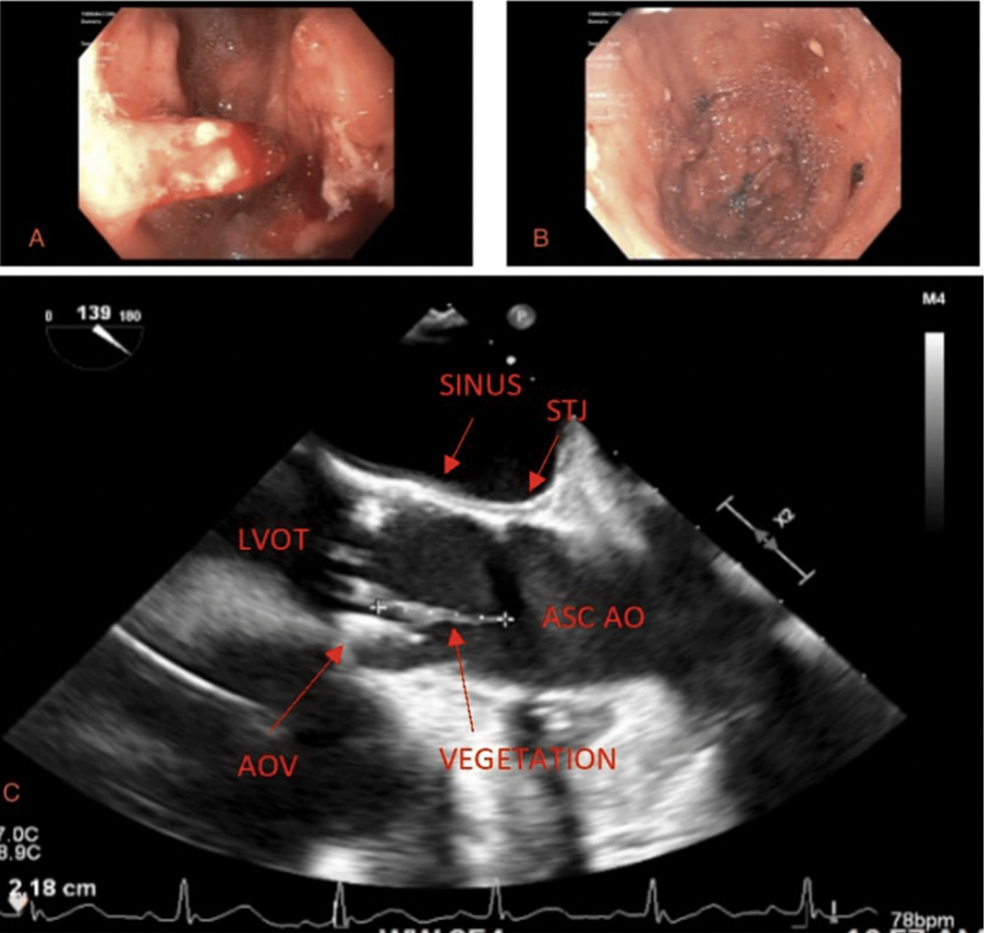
Endoscopic stomach views before and after argon plasma coagulation and subsequent vegetation on the aortic valve 1A) Pre-pyloric region with active bleeding from gastric antral vascular ectasia 1B) gastric antral vascular ectasia post-argon plasma coagulation. 1C) Transesophageal echocardiogram, mid-esophageal long-axis view at the level of the aortic valve showing a 2-centimeter vegetation coming off the right coronary cusp of the aortic valve.
